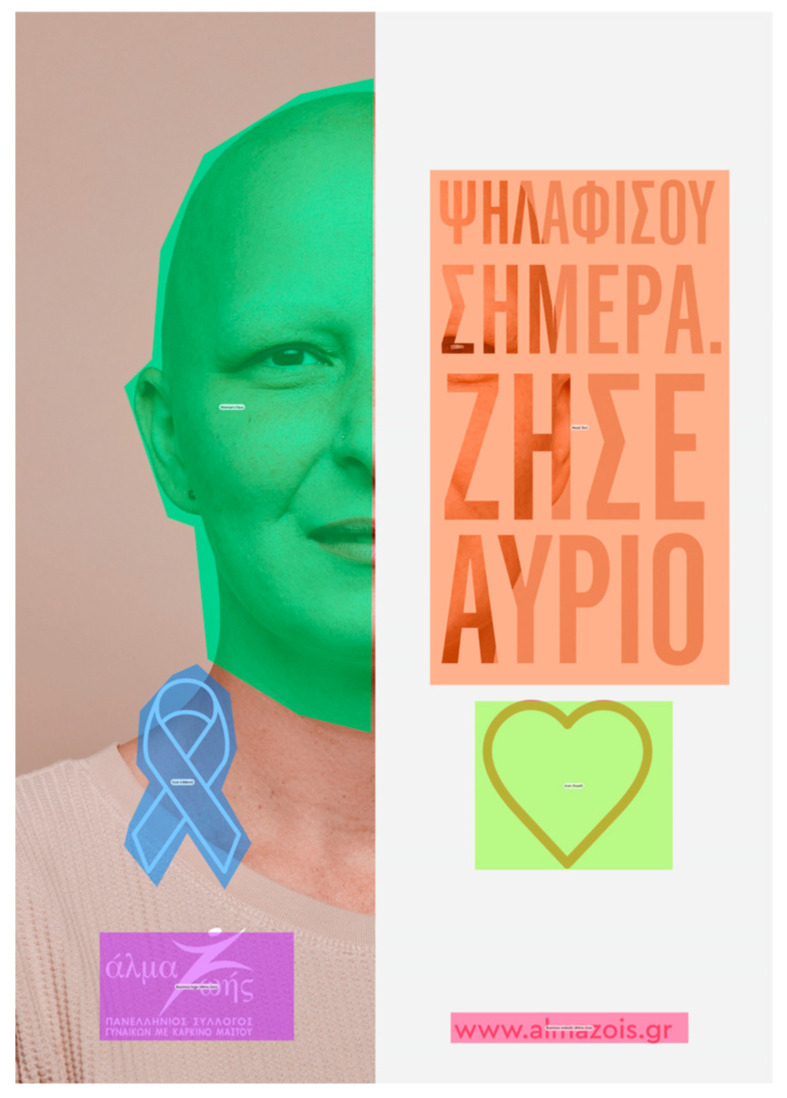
Example AOI delineation on a representative breast cancer awareness ad with a message of hope and survival after treatment (English translated caption: “Do a self-exam today. Live tomorrow.”). Colored polygons denote AOIs by category (Text, Image/Visual, Symbol, Logo, Website/CTA, and Source/Authority). Overlap priority is Website/CTA > Logo > Symbol > Source/Authority > Text > Image/Visual, ensuring that boundary fixations are assigned consistently. Polygons were drawn at native resolution in Tobii Studio and used for all AOI-based metrics (TTFF, fixation count, and total fixation duration).
